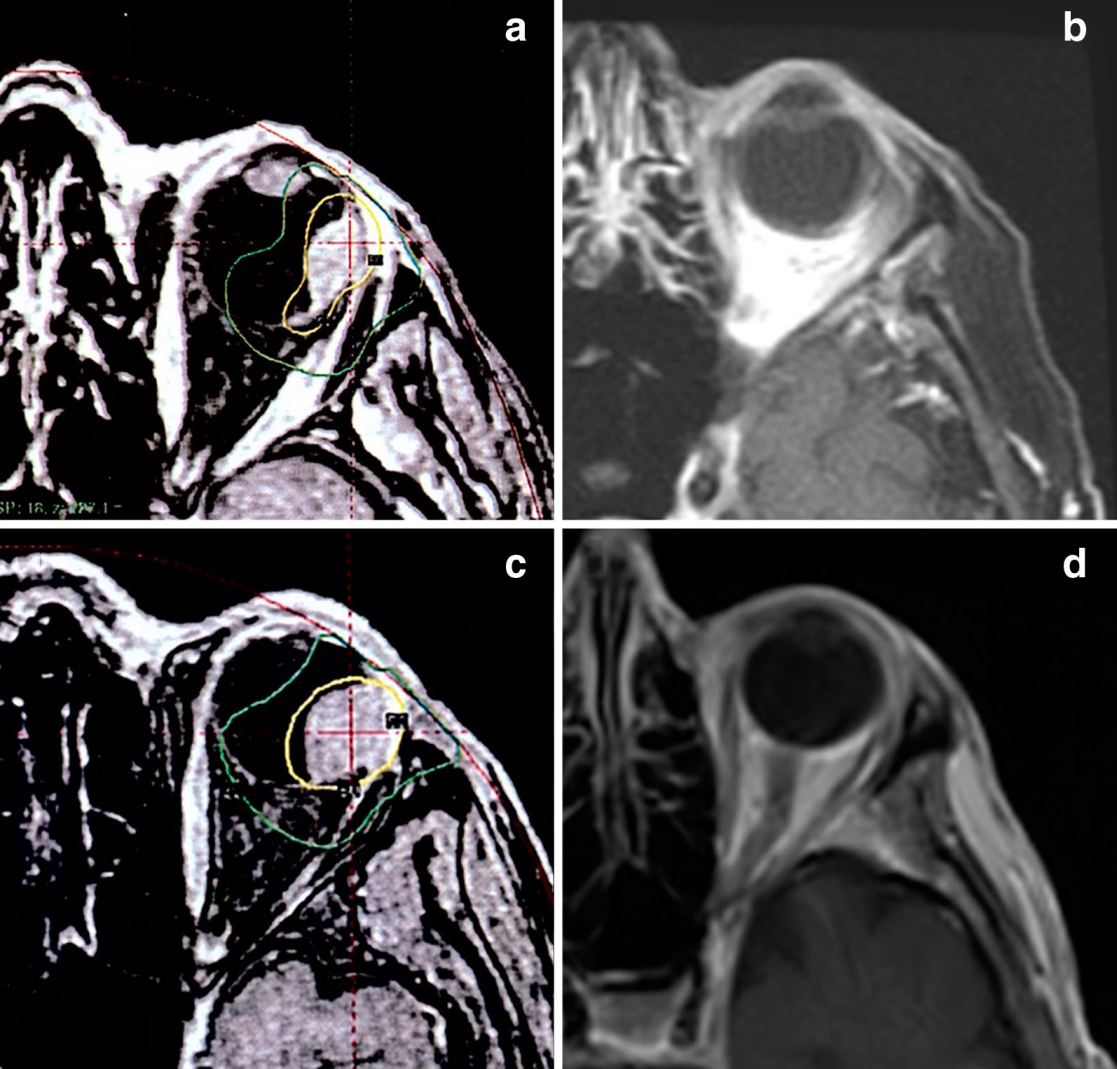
a Representative gamma knife planning MRI of a patient with choroidal melanoma treated with 18 Gy at the 50% isodose line. b gadolinium-enhanced T2 MRI of the orbit of the same patient depicted in tile a, after 7 months, shows interval decrease in size of choroidal mass. c Representative gamma knife planning MRI of a patient with choroidal melanoma treated with 27 Gy at the 50% isodose line. d Gadolinium-enhanced T2 fat saturation MRI of the orbit of the same patient depicted in tile c, after 48 months, shows interval decrease in size of choroidal mass
